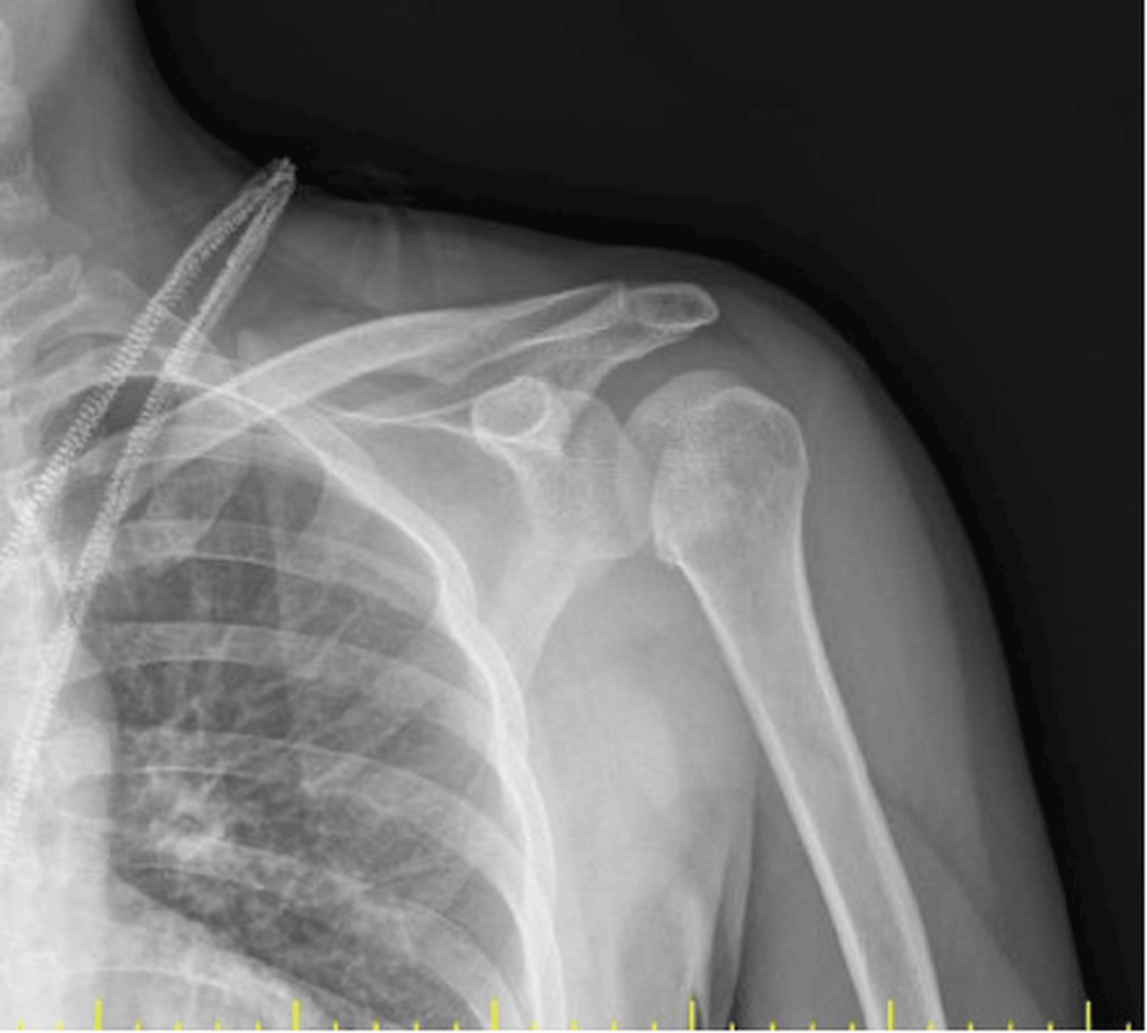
Left shoulder anterioposterior X-ray radiograph taken in the emergency department (Patient 1)
